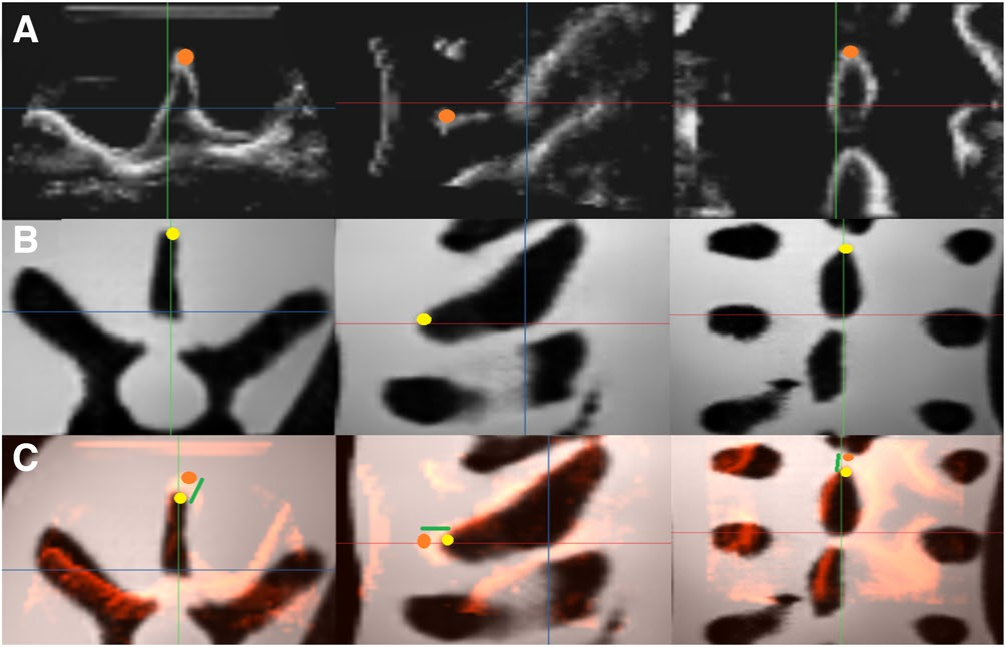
The clinical expert assesses the algorithm’s volume registration accuracy by qualitatively analyzing the spatial alignment between the input US volume and its corresponding generated MRI volume (row C, green line segment) using the target registration error. This evaluation offers additional quantitative insights, focusing on the anatomical characteristics of the thoracic vertebrae present in both volumes. The images depict the input US volume (row A, orange dot) and its corresponding generated MRI volume (row B, yellow dot) along the transverse, sagittal, and coronal planes, arranged from left to right.
